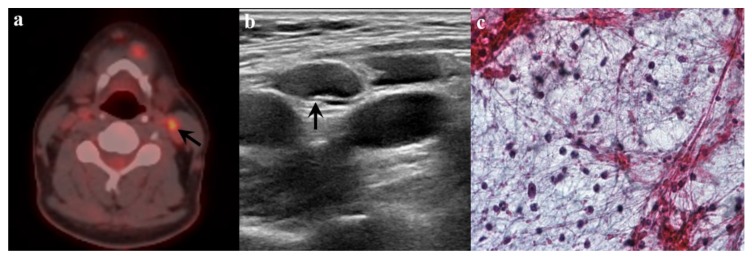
A 52-year-old man with tongue cancer. (a) PET/CT shows focal areas of increased fluorodeoxyglucose uptake with a maximum standardized uptake value of 5.9 in the left level II. (b) Ultrasonography revealed a benign-looking lymph node with normal cortical echogenicity, ovoid shape, preservation of fatty hilum and a short axis diameter of 0.6 cm. (c) Ultrasonography-guided fine needle aspiration was performed, and cytologic examination showed benign lymphoid cells scattered in the granular background. Epithelial cell clusters for suspicious malignancy were not found.
